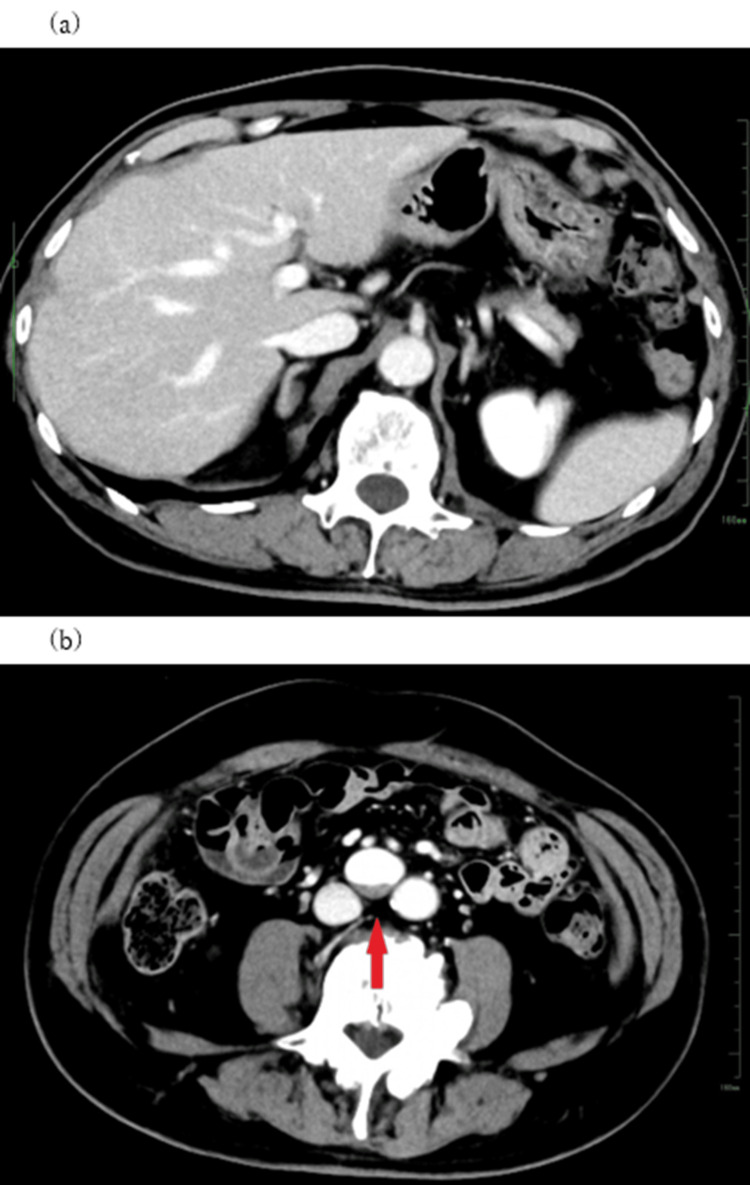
Axial view of the enhanced abdominal CT at first detailed examination. (a) No embolus of the intrahepatic portal vein is revealed. (b) Intraluminal thrombus is visualized in the superior mesenteric vein (SMV) aneurysm (arrow).
